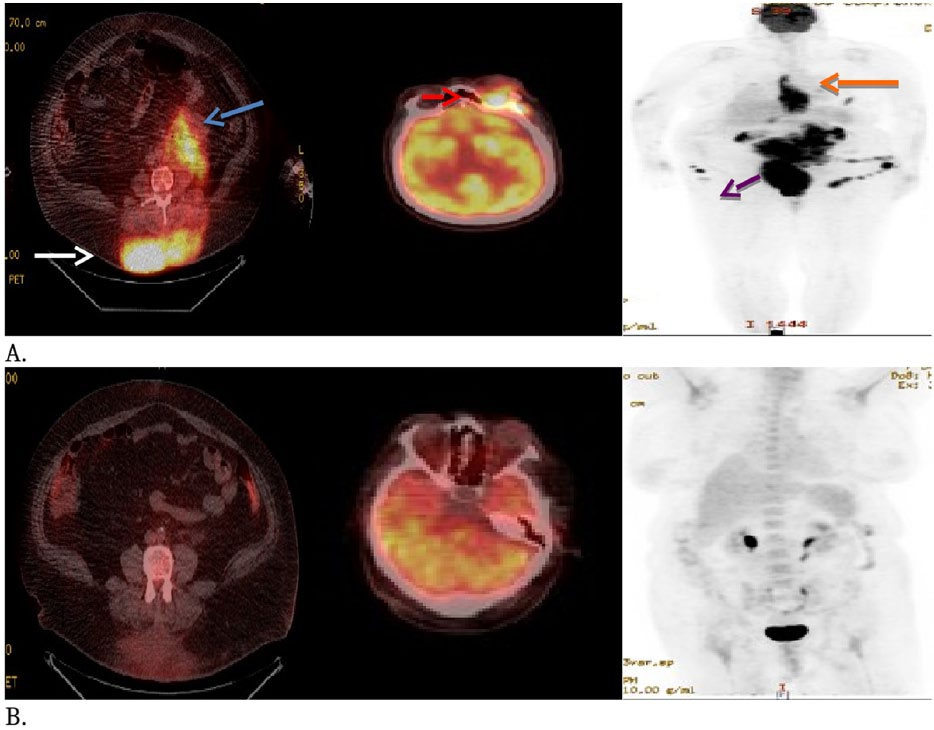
PET/CT. (A) Hypermetabolic orbital mass (red arrow), posterior mediastinal mass (orange arrow), retroperitoneal mass (blue arrow), mass within the midline lumbar subcutaneous tissues (white arrow), diffuse hypermetabolic activity between the bladder and rectum that corresponds to the uterus (purple arrow). (B) Complete resolution of hypermetabolic soft tissue activity.
